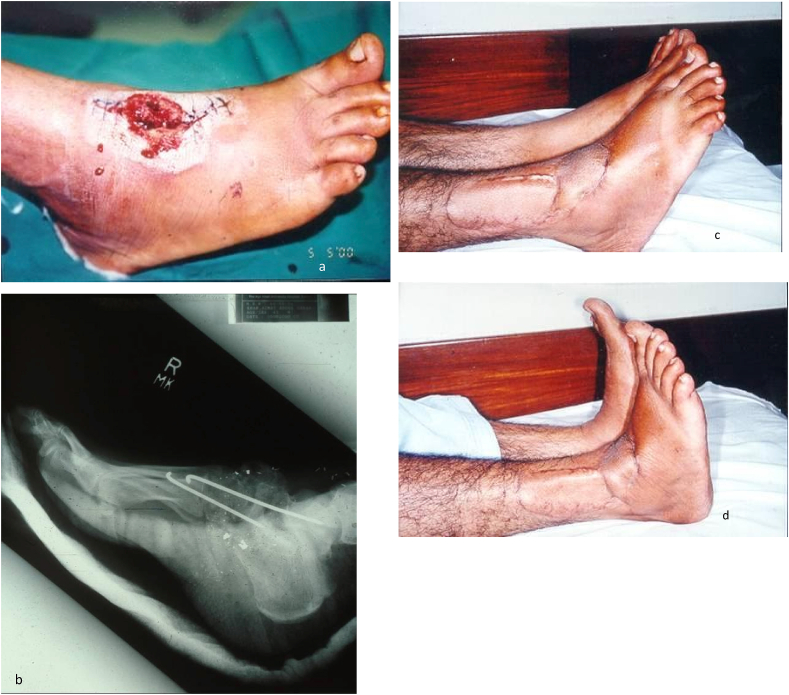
40 years old gentle man had gunshot injury to right foot with grade III open fracture of tarsometatarsal joints (Fig 3.a). Following debridement and skeletal stabilization, as shown in xrays (Fig 3.b). The defect was covered with Supramalleolar flap with good plantar and dorsiflexion (fig 3.c and 3.d).
